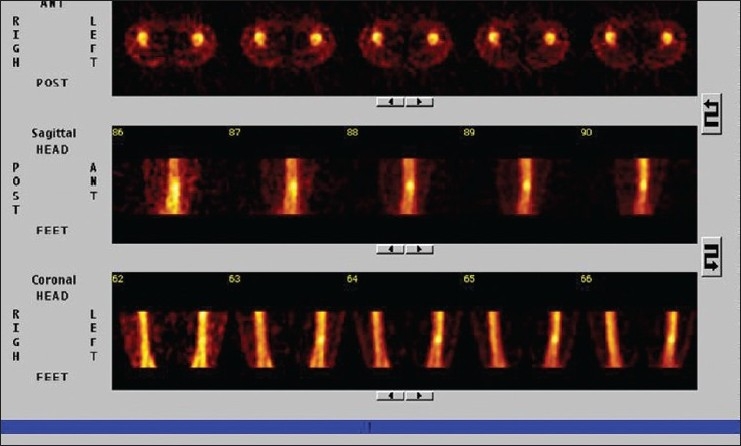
Bone SPECT showing focal uptake of the nidus
